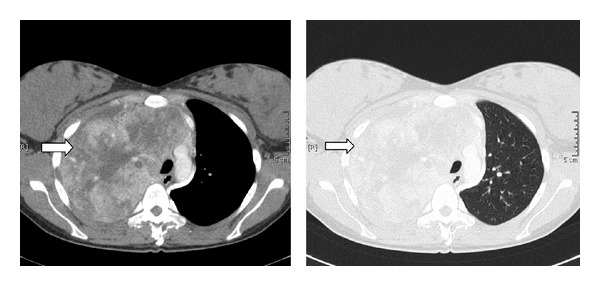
Contrast-enhanced computed tomography scan of a large tumor mass in the right upper lobe with extensive mediastinal infiltration.
